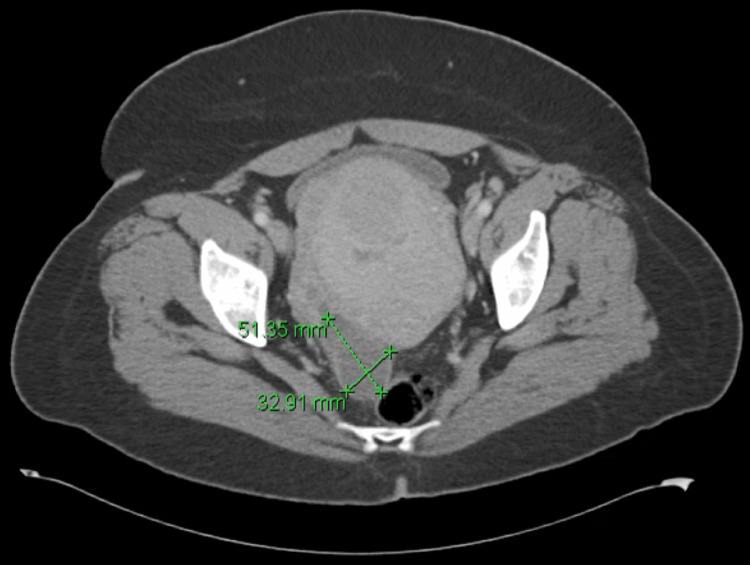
The patient's CT abdomen and pelvis at admission shows a right posterior pelvic fluid collection measuring 32.91 mm x 51.53 mm consistent with a TOA. CT: Computed tomography; TOA: Tubo-ovarian abscess
